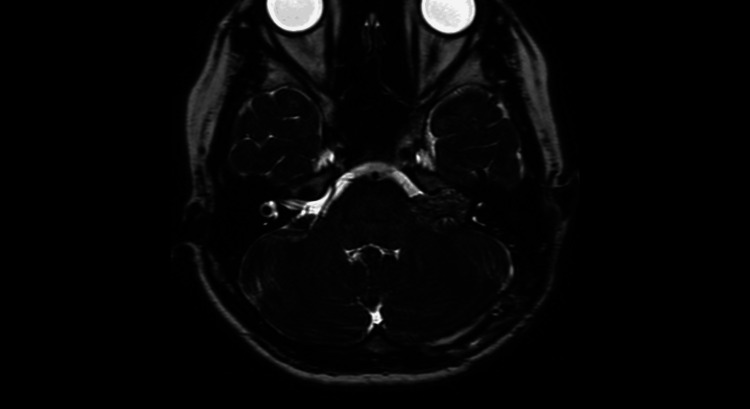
Postoperative MRI T2-weighted image Residual tumor was noted, but the compressive effect on the brainstem and cerebellum had been reduced.
